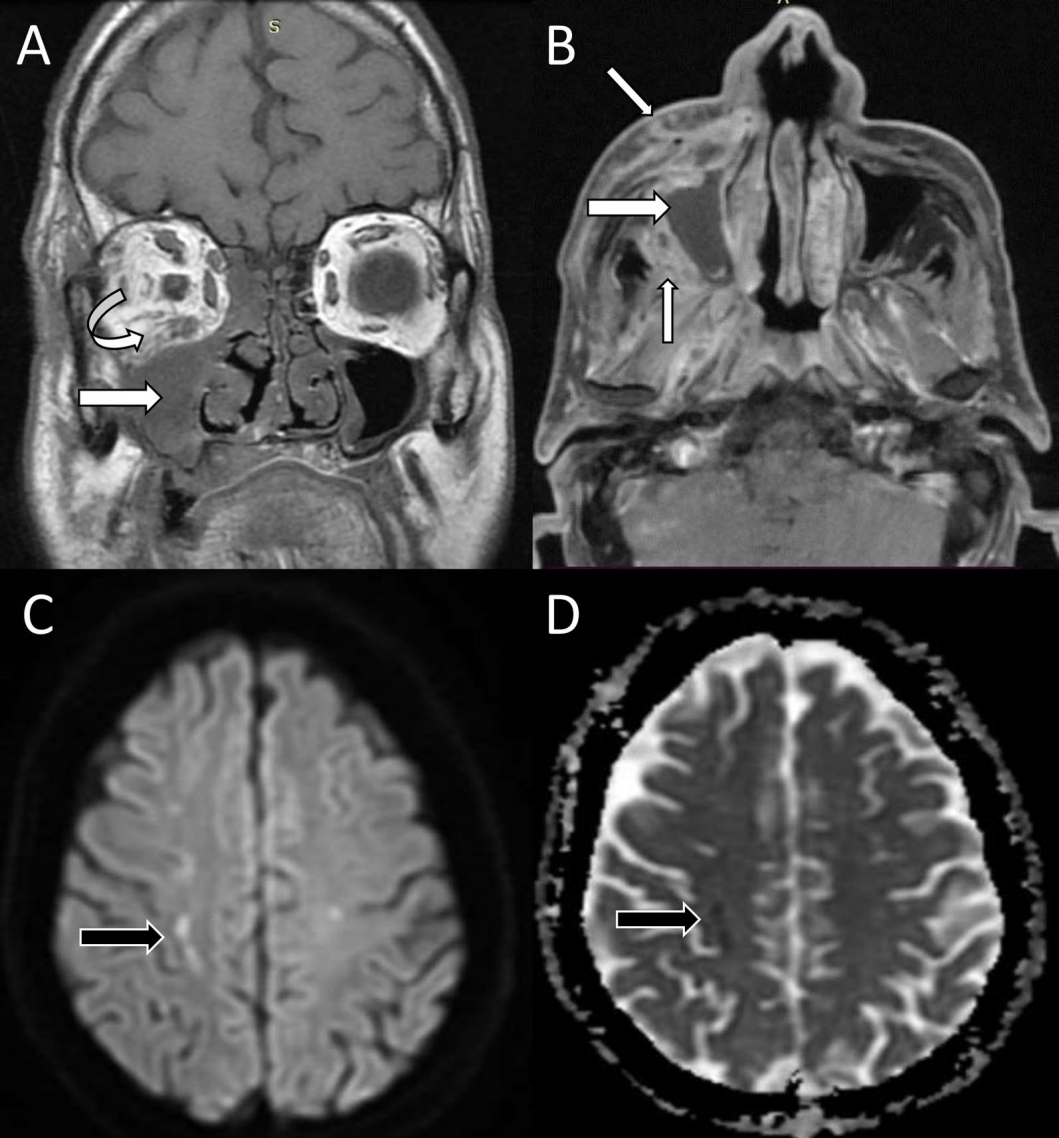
Rhinocerebral mucormycosis-associated cerebral infarct. Coronal T1-weighted image (A) shows soft tissue in the right nasal cavity and maxillary sinus (white arrow) with intraorbital fat stranding (curved arrow). Contrast-enhanced axial T1-weighted fat-saturated image (B) shows non-enhancing maxillary sinus lesion (large white arrow) with the heterogeneous enhancement of the periantral soft tissues (small white arrows). Diffusion-weighted and apparent diffusion coefficient (ADC) images (C, D) show foci of diffusion restriction with corresponding low ADC signal (black arrows) in the right centrum semiovale in the watershed territory
